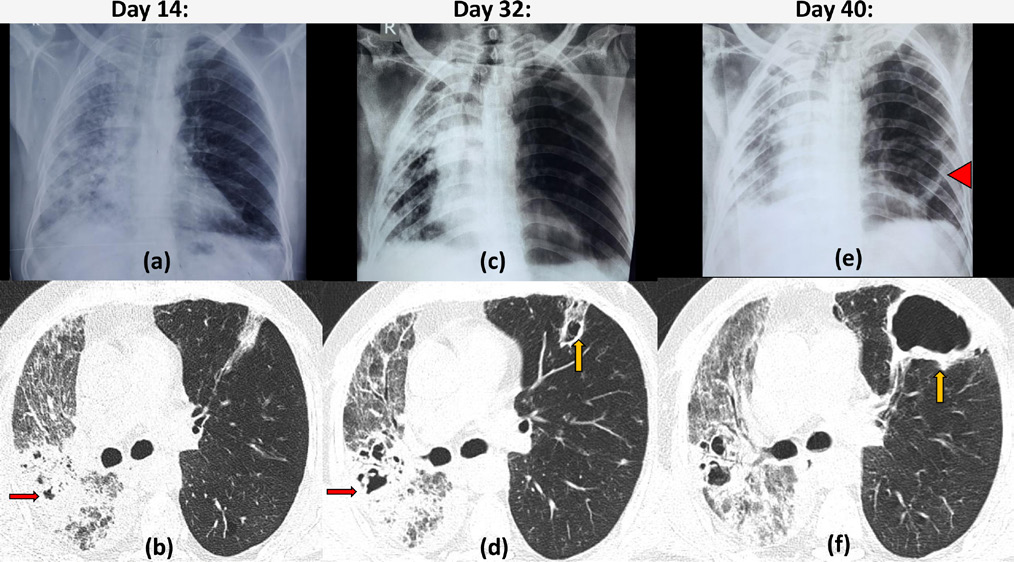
Bacterial coinfection with COVID19 infection: 50-year-old diabetic male with chest tightness and dyspnoea since 10 days, tested positive for COVID19. Imaging on Day 14: frontal chest radiograph (a) revealed marked asymmetrical involvement with infiltrates in the entire right lung and relative sparing of the left lung. HRCT axial section (b) revealed patchy consolidation with few tiny cystic areas within (red arrow). Associated interlobular septal thickening and mild volume loss of right lung was also present. Imaging on Day 32: patient had only slight clinical improvement chest radiograph (c) shows marked ipsilateral mediastinal shift and volume loss with some resolution of infiltrates. HRCT (d) revealed increased size and number of the areas of cavitation which can be seen bilaterally now in areas of previous consolidation (yellow arrow) and were assumed to be a sequelae of COVID19. Imaging on Day 40: patient had clinically worsened Chest radiograph (e) revealed a large cavity in the left lower zone (arrowhead). HRCT (f) revealed marked increase in the size of the cavity in the lingular segment showing thick irregular walls (yellow arrow), disproportionate to the area of previous consolidation, a superinfection was suspected. The features in the right lung remained unchanged. On sputum culture, *Klebsiella pneumoniae* was isolated.
